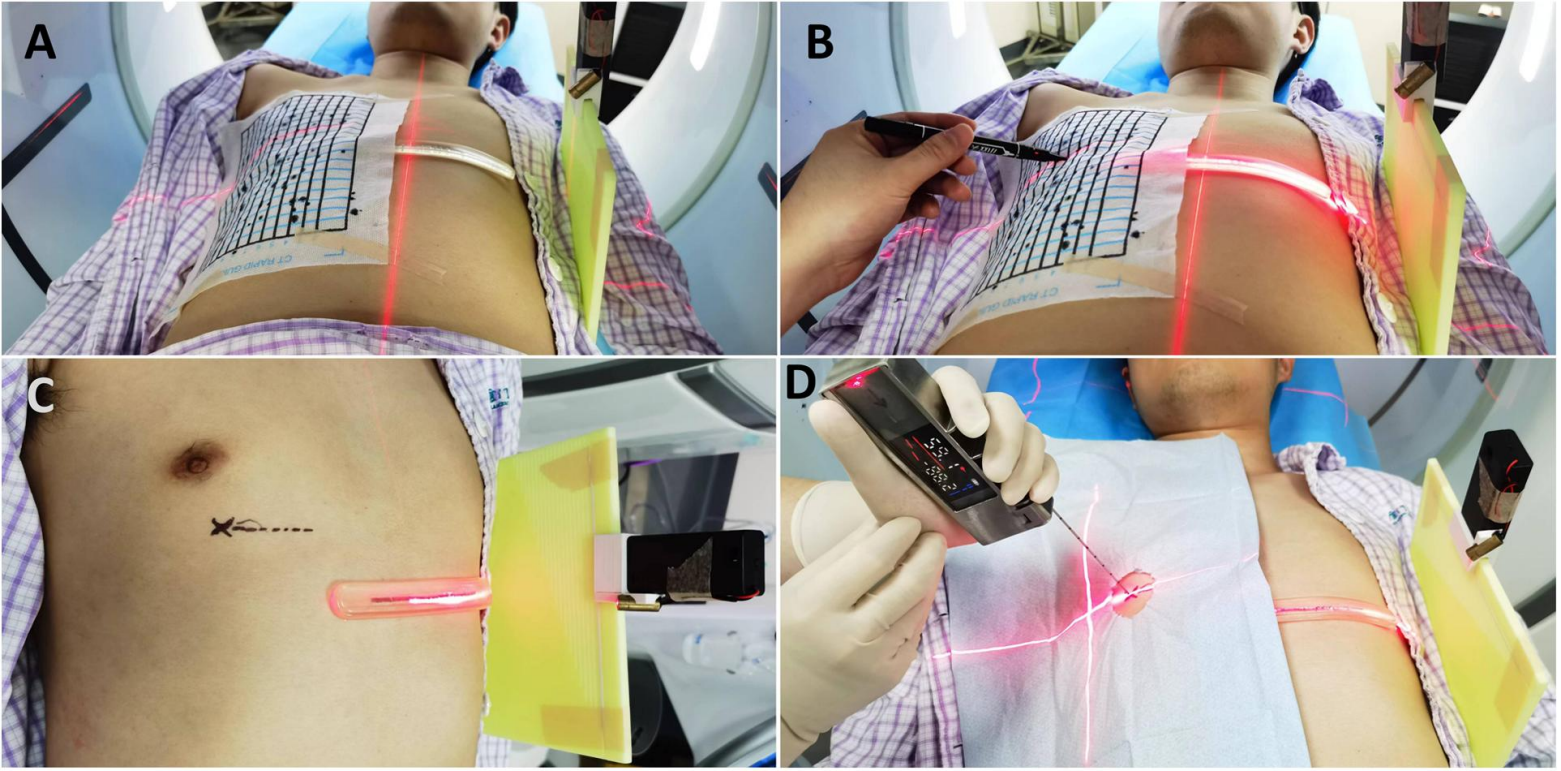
Percutaneous lung puncture by matching thoracic respiratory activity combined with laser angle guidance. (A) After the thoracic activity calibration plate and positioning paper are properly placed, the first ct scan is performed. (B,C) The patient is instructed to adjust the breathing amplitude to make the laser line coincide with the indicator band. At this time, the horizontal line corresponding to the puncture level and the intended puncture point are marked on the patient's skin according to the laser indication of the CT machine. (D) The patient is once again instructed to adjust the breathing amplitude to make the laser line projected by the positioning buckle of the thoracic activity calibration plate coincide with the indicator band. The laser angle guider is pushed and the puncture needle is advanced to the predetermined depth.
